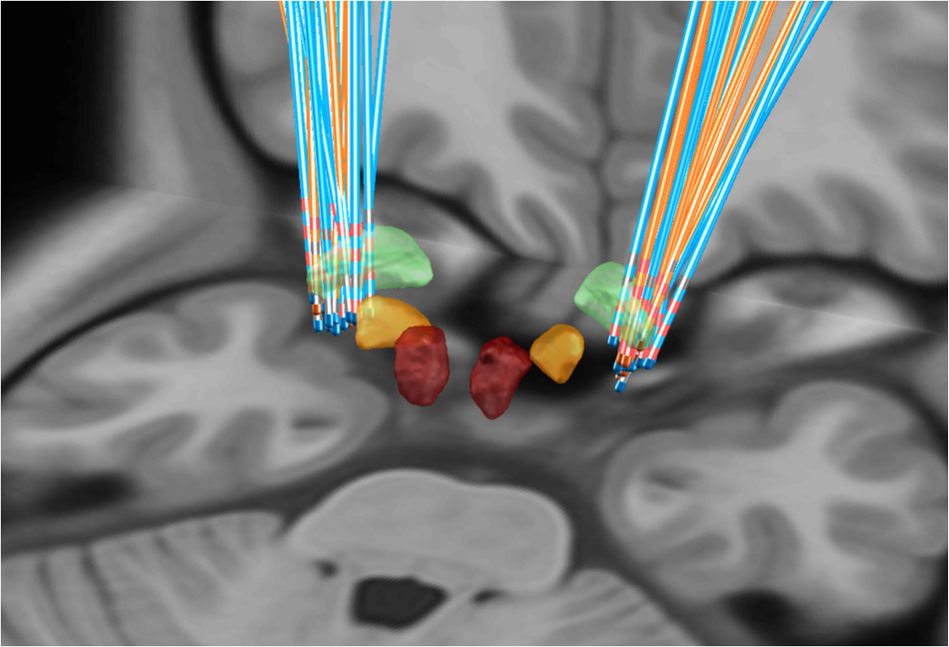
Reconstruction of the DBS electrodes. The electrodes of 10 patients with camptocormia (marked in orange) and 18 patients without camptorcormia (maked in blue) were shown on the T1-weighted Montreal Neurological Institute (MNI) template. Active contacts were marked in red. Masses with yellow described the location of the STN, red for the red nucleus, and green for the GPi.
